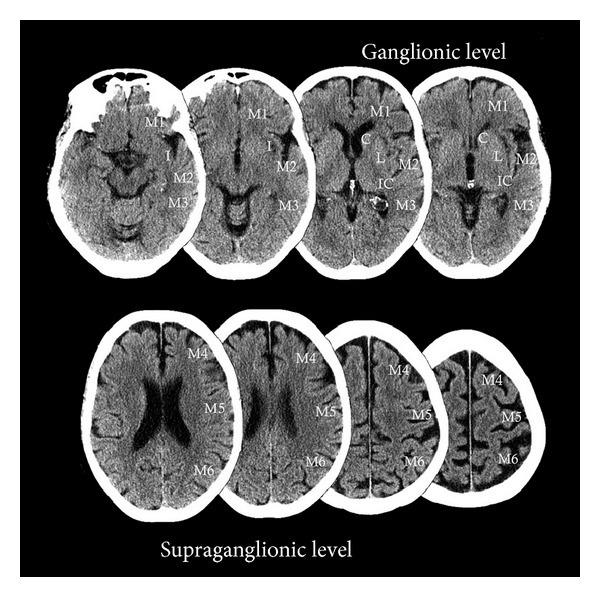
Obtained from http://www.aspectsinstroke.com/, demonstrating the ASPECTS scoring methodology, axial NCCT images showing the MCA territory regions as defined by ASPECTS. C, caudate, I, insularribbon, IC, internal Capsule, L, lentiform nucleus, M1, anterior MCAcortex, M2, MCA cortex lateral to the insular ribbon, M3, posteriorMCA cortex, M4, M5, M6 are the anterior, lateral, and posterior MCAterritories immediately superior to M1, M2, and M3, rostral to basal ganglia. Subcortical structures are allotted 3 points (C, L, and IC). MCA cortex is allotted 7 points (insular cortex, M1, M2, M3, M4, M5, and M6). (Reprint permission obtained from Dr. Mayank Goyal, professor of radiology and clinical neurosciences, * *Foothills Medical Centre, * *University of Calgary).
